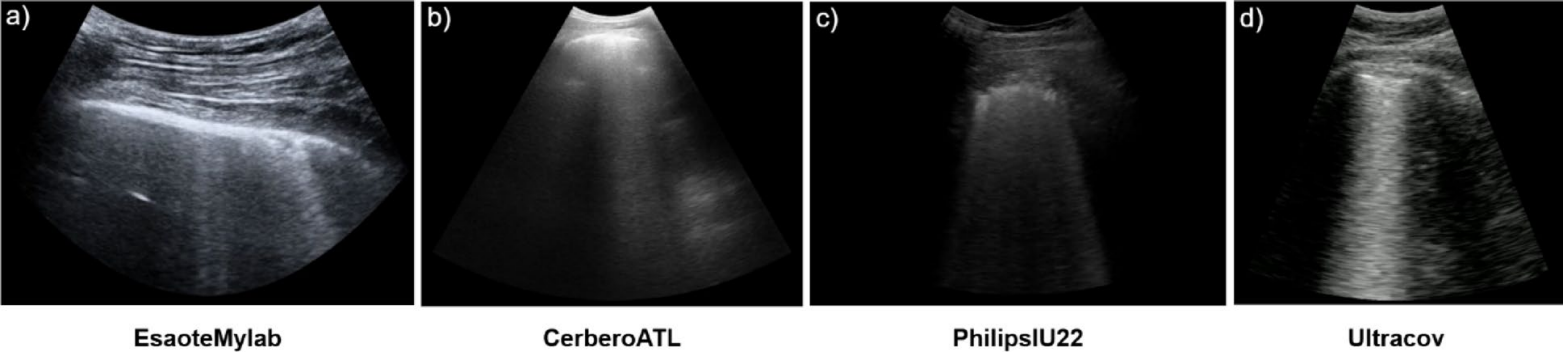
Comparison of LUS image quality across different ultrasound scanners
